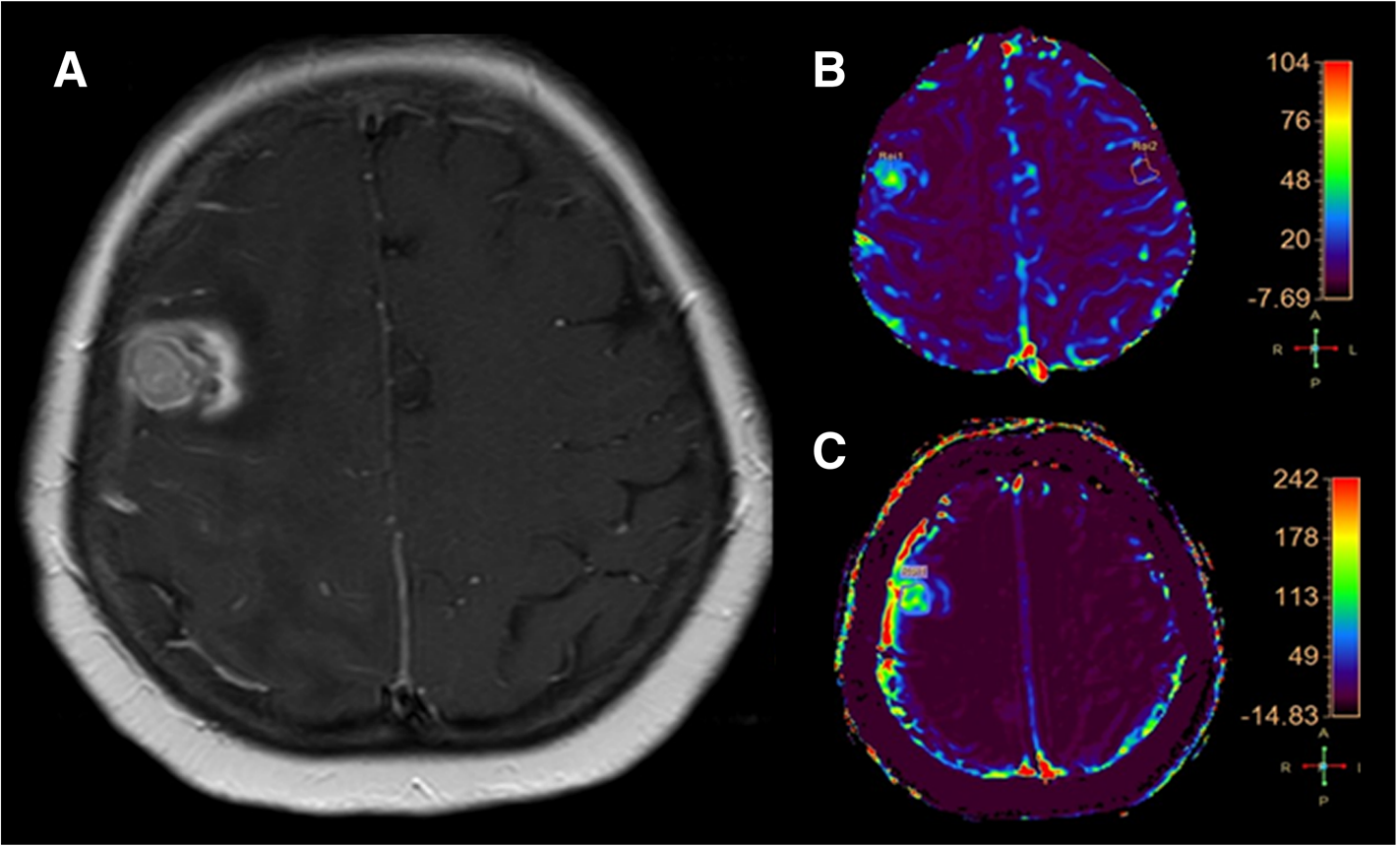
a (axial SE T1-weighted after Gadolinium administration), b (rCBV colorimetric map), c (Ktrans colorimetric map). Right frontal breast metastasis treated by SRS follow-up MRI examination. The enhancing lesion shows a central component with high rCBV and Ktrans values according to tumor recurrence and a peripheral component with low rCBV and Krans values according to the coexistence of radiation necrosis
